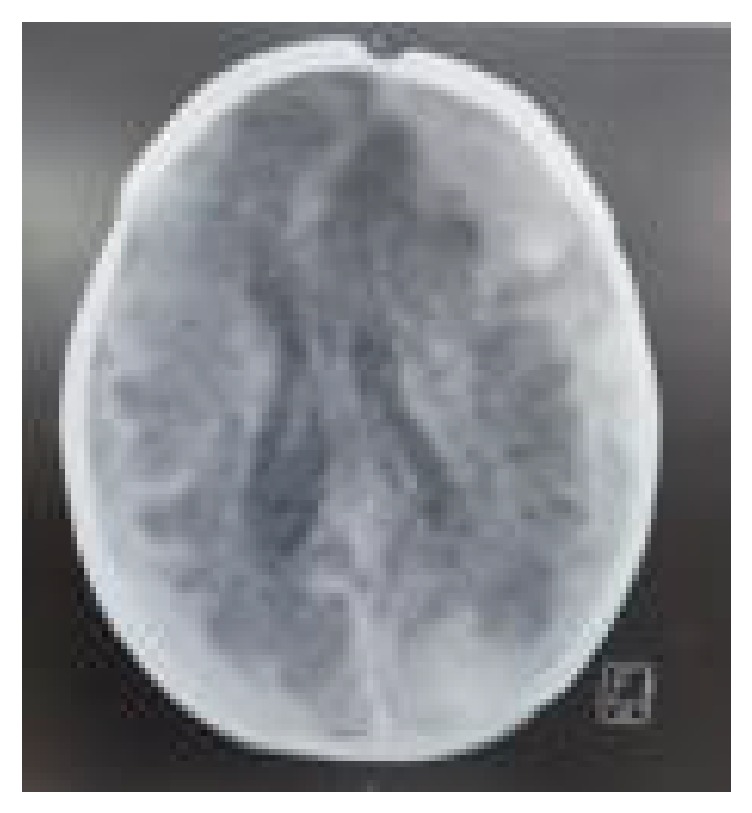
Cranial CT of the newborn. Cranial CT scan of the newborn showed bilateral intraventricular hemorrhage with ventricular dilatation and midline shift.
